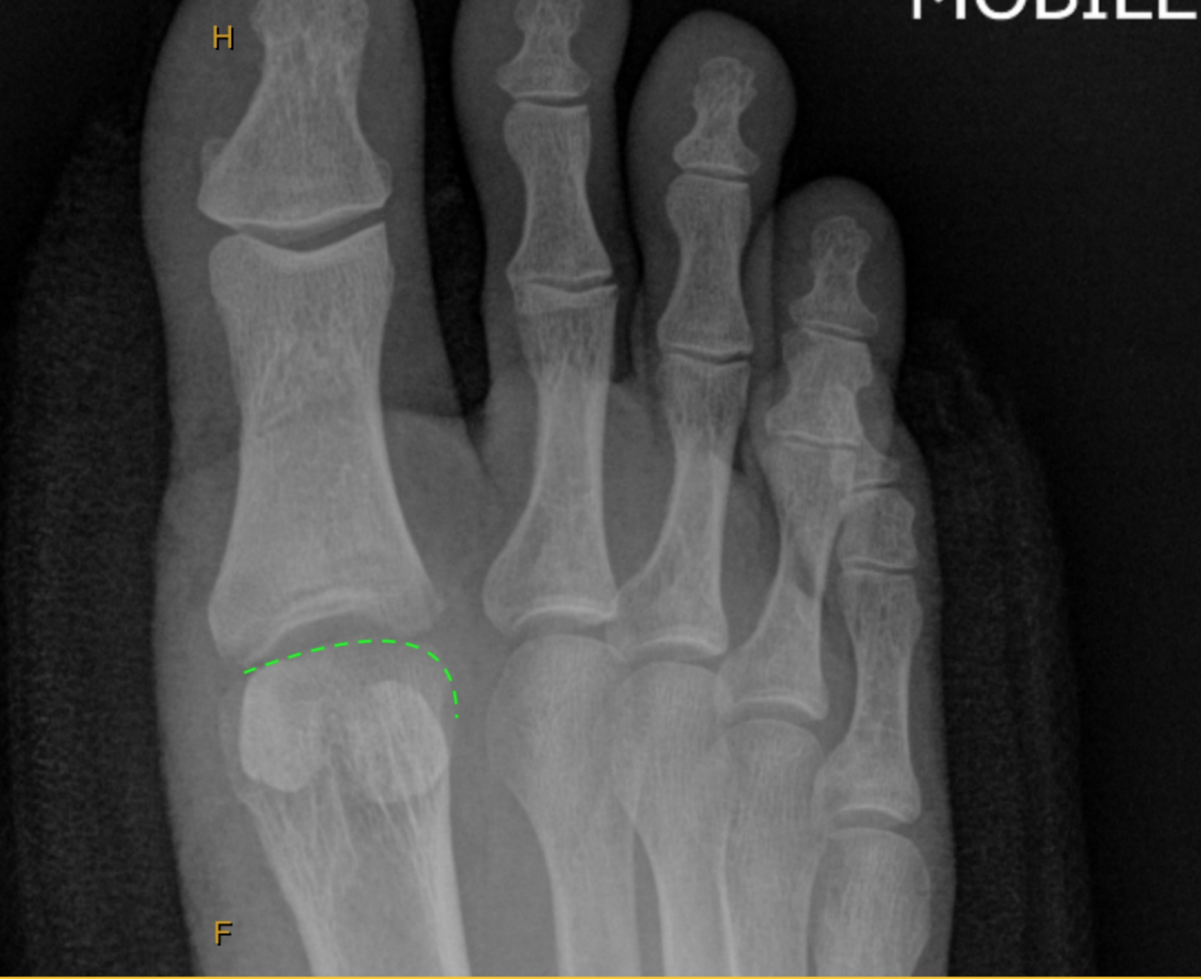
Immediate postoperative anterior/posterior radiograph showing normal metatarsal head morphology when compared to the preoperative image.
